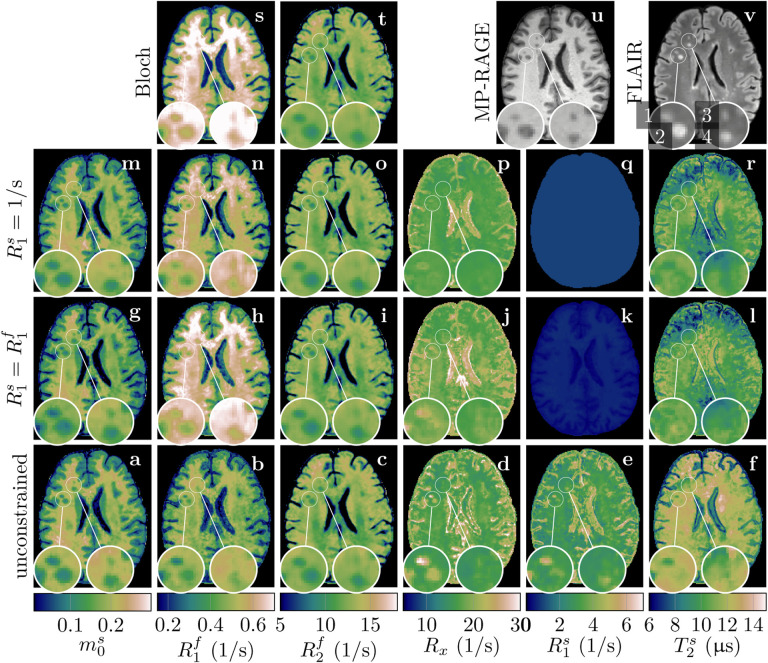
Quantitative MT maps of an individual with MS fitted with the proposed unconstrained MT model (a-f), MT model constrained by $R_{1}^{s}=R_{1}^{s}$ (g-l) and $R_{1}^{s}=1/\text{s}$ (m-r), and the Bloch model (s,t). Note that k is a replica of h on a different color scale. MP-RAGE (u) and FLAIR (v) scans are provided for reference. The magnifications highlight four lesions (labeled in v) that have similar appearances on the FLAIR and MP-RAGE and reveal heterogeneity on the qMT maps, heterogeneity that is most pronounced in the unconstrained qMT maps.
